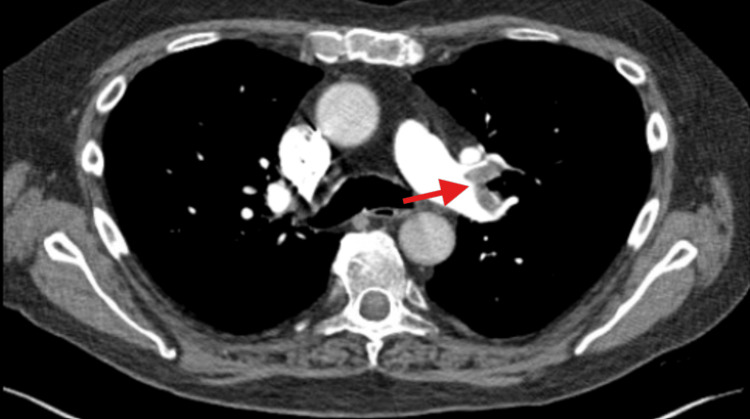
CT angiogram scan of the chest demonstrating a large left main pulmonary artery embolism (red arrow) CT: computed tomography.
